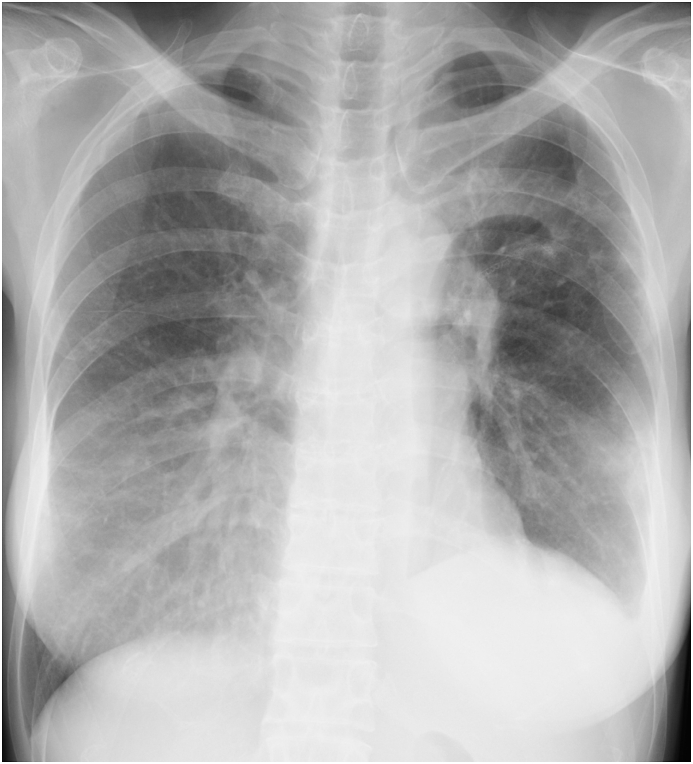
Chest radiography during the previous hospital admission.
